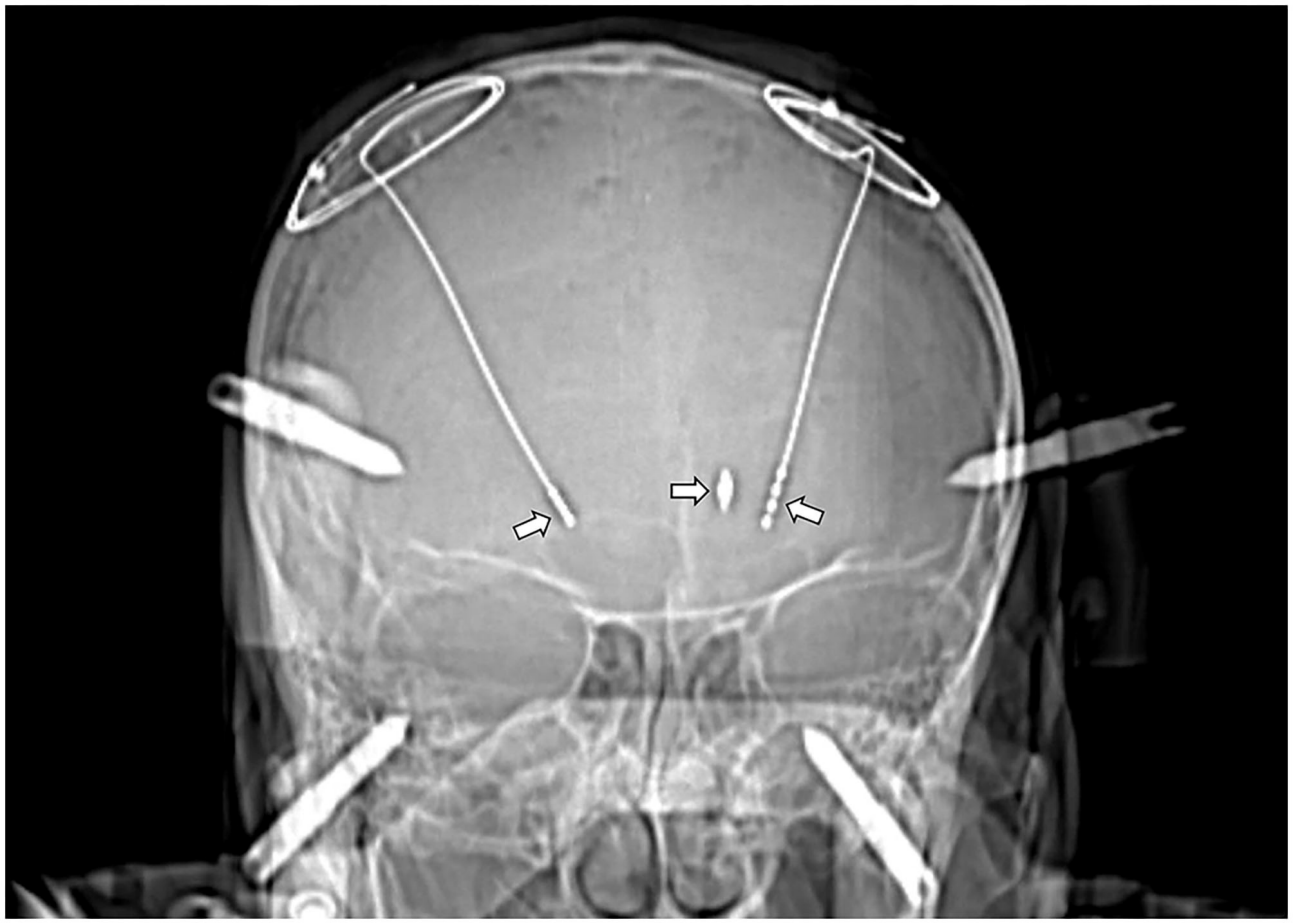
CT image after the DBS implantation (left arrow: STN-DBS implantation in the right cerebral hemisphere; right arrow: GPi-DBS implantation in the left cerebral hemisphere; mid arrow: The bullet was located on the left STN electrode trajectories).
